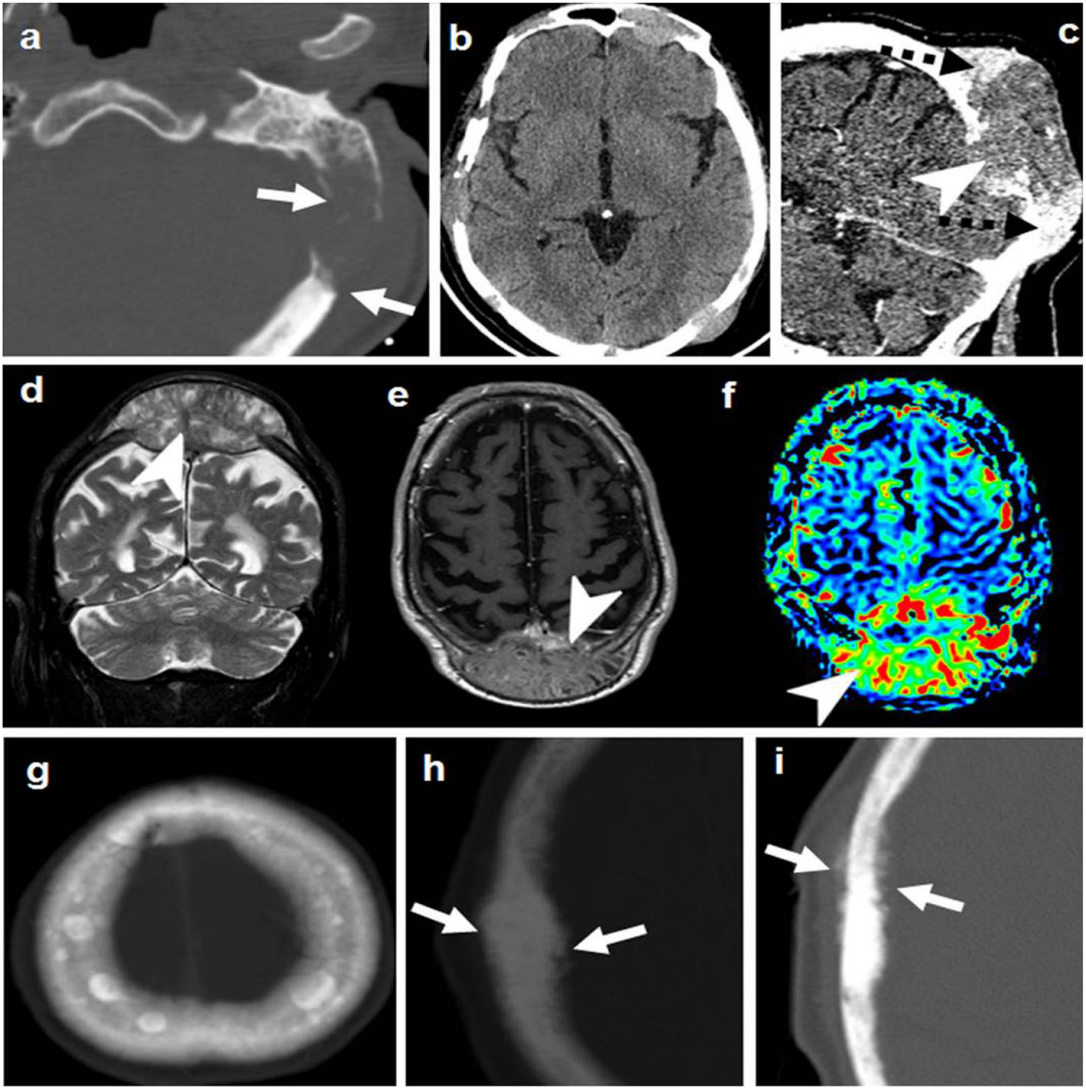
Metastases. Lytic metastases (a–f). CT of three patients (a–c) shows: a Solitary with narrow zone of transition (arrows), b multiple with slight soft-tissue components, and (c) solitary, giant, with mixed necrotic (arrowhead) and hypervascular (dashed arrows) soft tissue. Another case (d–f) with T2WI, T1WI post-contrast, and DSC-PWI shows solitary, giant, solid-enhancing, and hypervascular soft-tissue component (arrowheads). g–i Three cases of blastic metastases. Multiple (g). Aggressive periosteal reaction (arrows in h–i)
